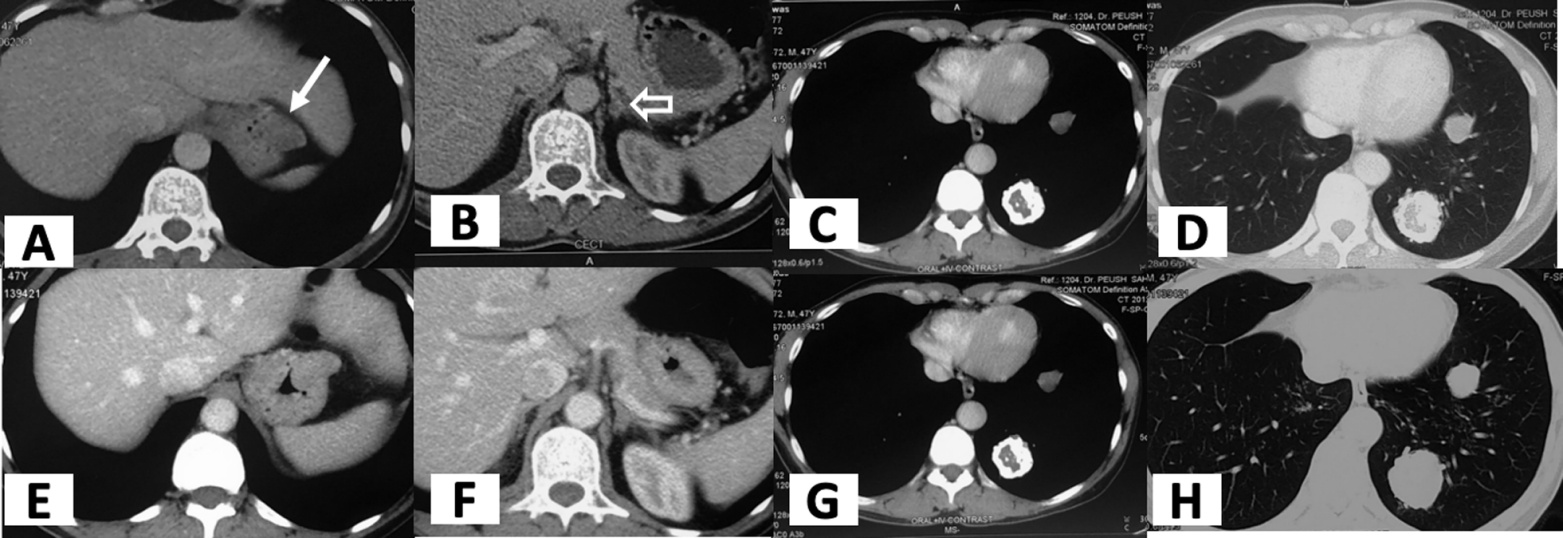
Axial CT showing exophytic gastric fundus mass (marked by arrow) (A), left adrenal nodule (marked by arrow) (B), and pulmonary calcified nodules/fibroma (C, D). On repeat scan, no significant change seen in gastric mass (E) and lung nodules (G, H), and left adrenal nodule has slightly reduced in size (F)
